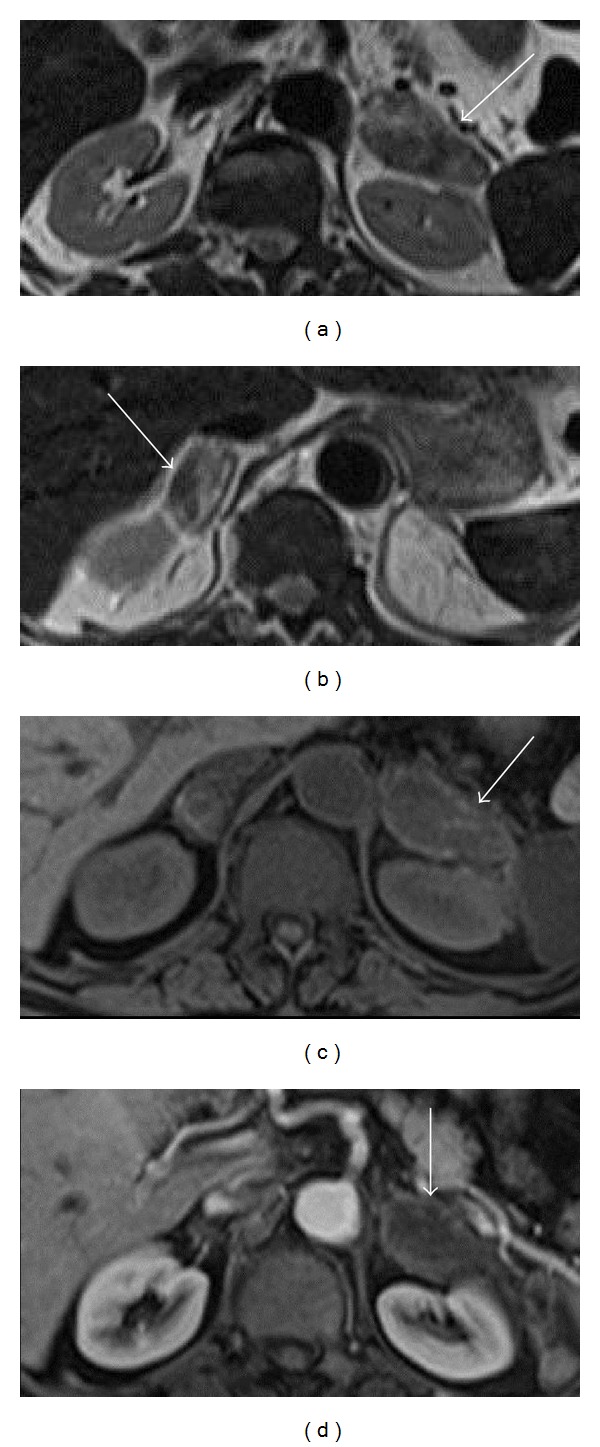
Bilateral enlarged adrenal glands with high signal intensity on axial T2- ((a), (b)) and T1-weighted (c) MRI images obtained before administration of gadolinium. Axial T1-weighted images (d) obtained after contrast injection reveal hyperintense masses with mild heterogeneous enhancement in hepatic arterial dominant phase.
